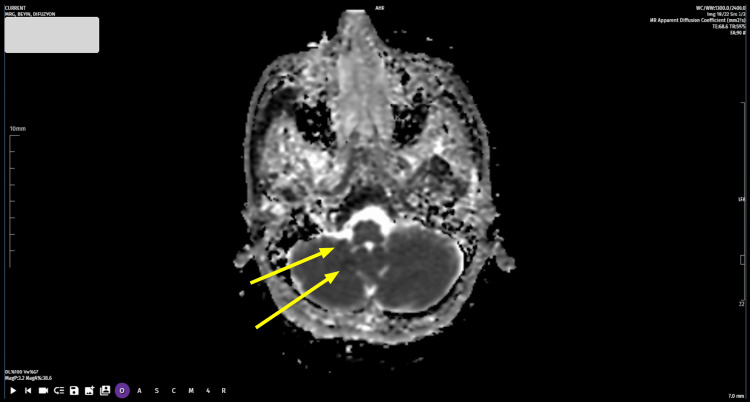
Apparent diffusion coefficient MRI of the brain The arrows point to the hypointense areas, which are consistent with an acute ischemic infarct in the distribution of the right vertebral artery.
